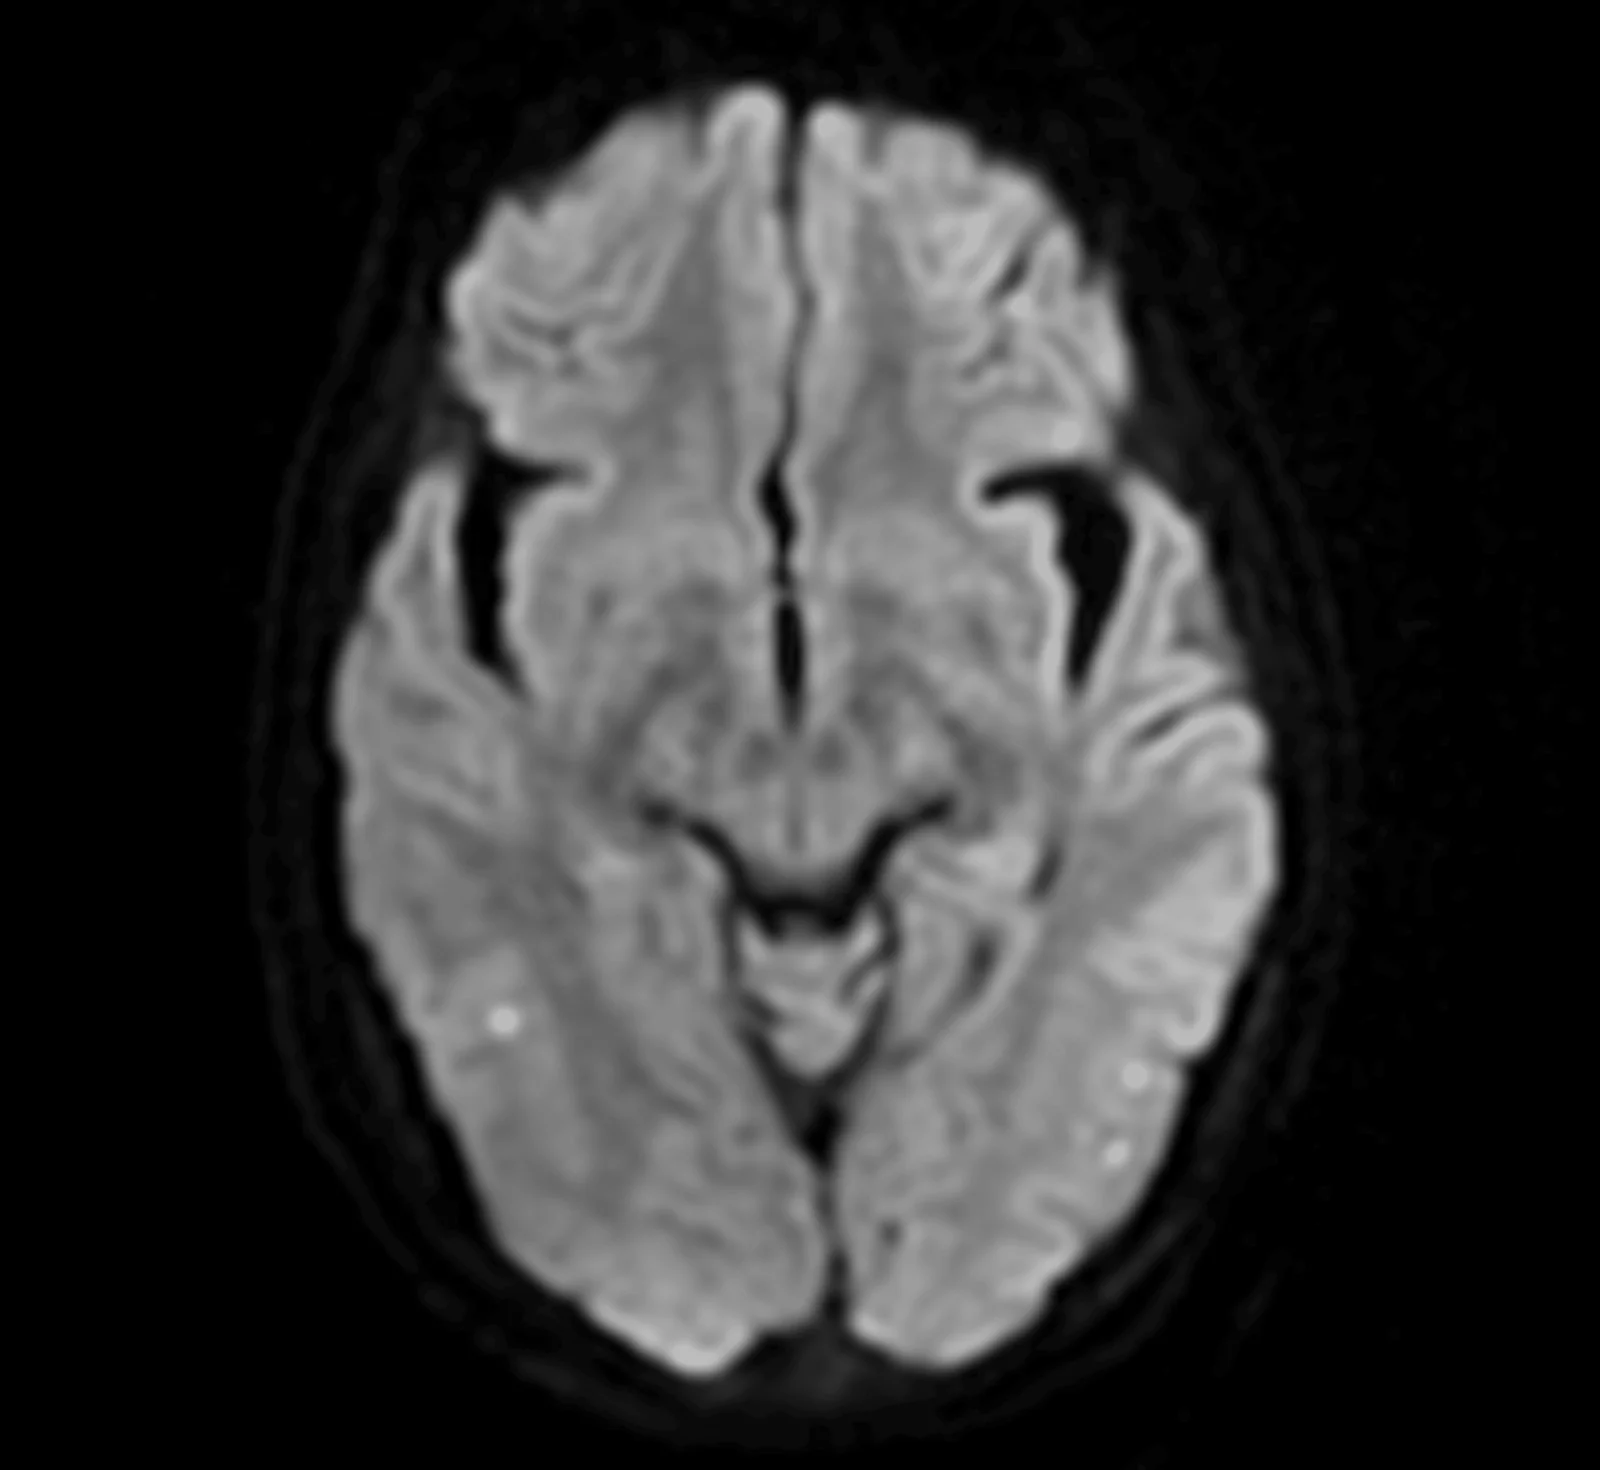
Magnetic resonance imaging of the brain (diffusion-weighted imaging) showing multiple acute lacunar infarcts consistent with small-vessel ischemic injury
